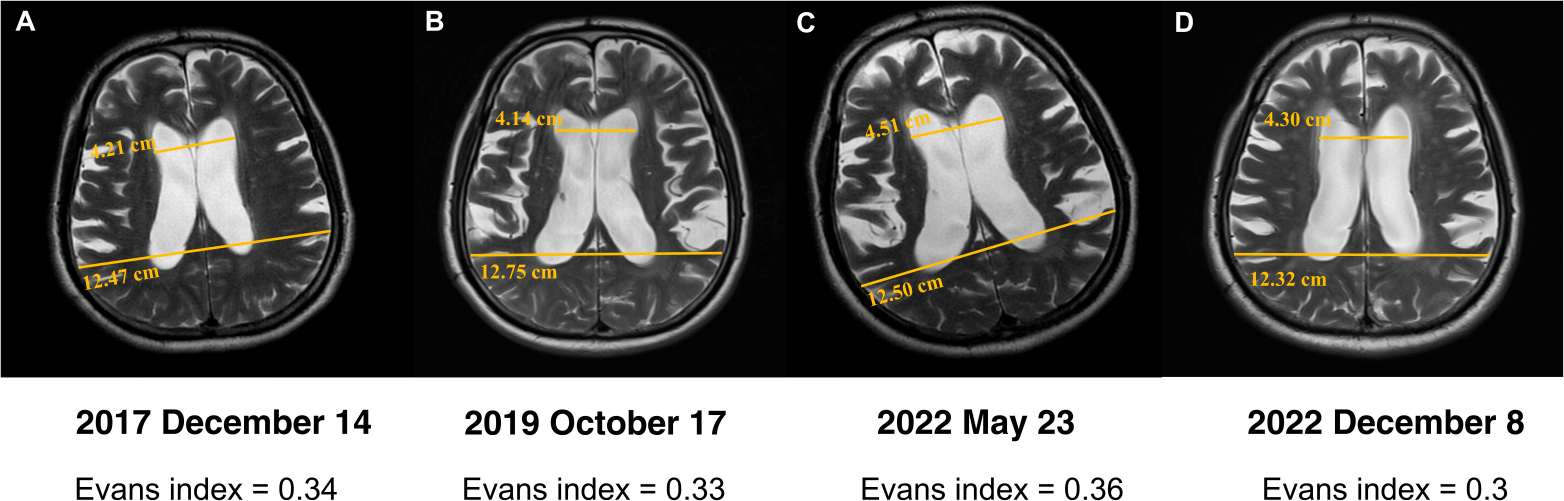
MRI images of a patient with iNPH in chronological order over a 4-year period. Evans index is shown in the picture to demonstrate extent of ventricular enlargement.
